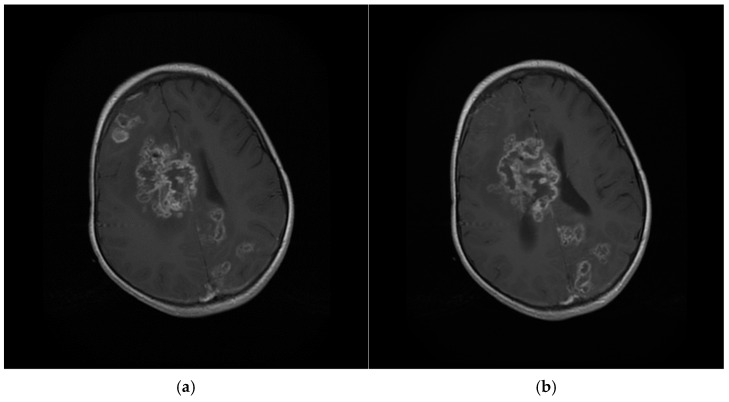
Brain MRI ((a,b): different slices), day + 183 post-2nd HCT.
